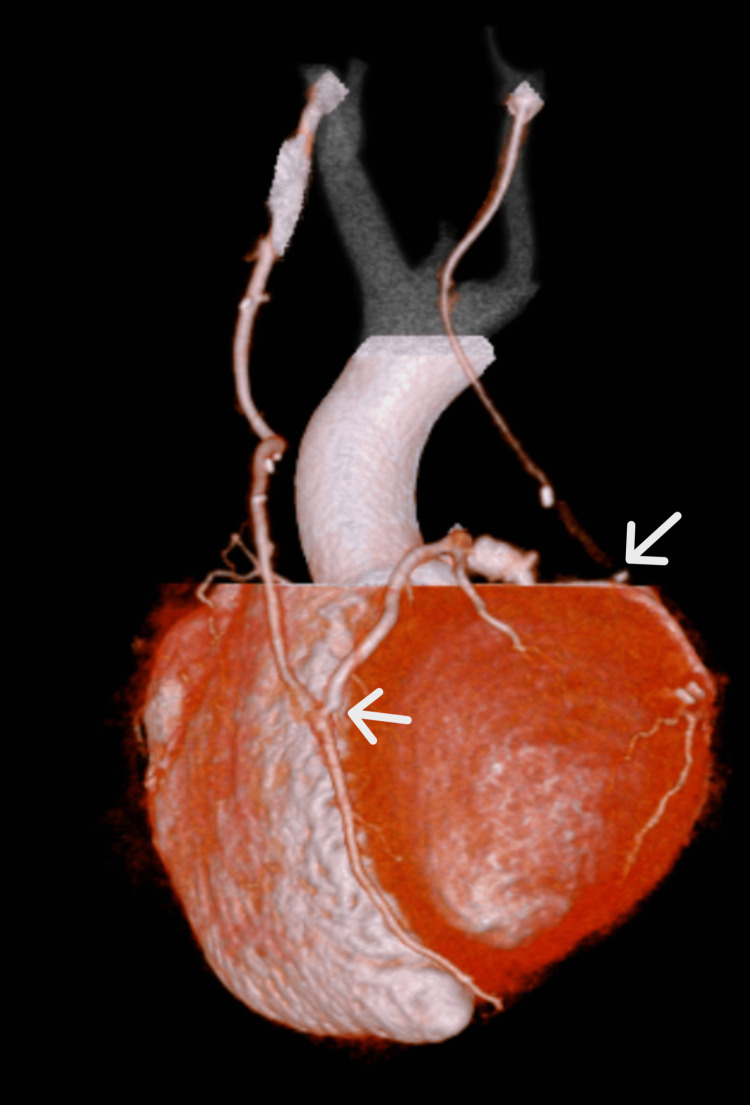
A reconstructed image from contrast-enhanced computed tomography after coronary artery bypass graft Bypass grafting has been performed from the right internal mammary artery to the LAD and from the left internal mammary artery to the LCX, with good patency of the vessels LAD, anterior descending artery; LCX, left circumflex artery
